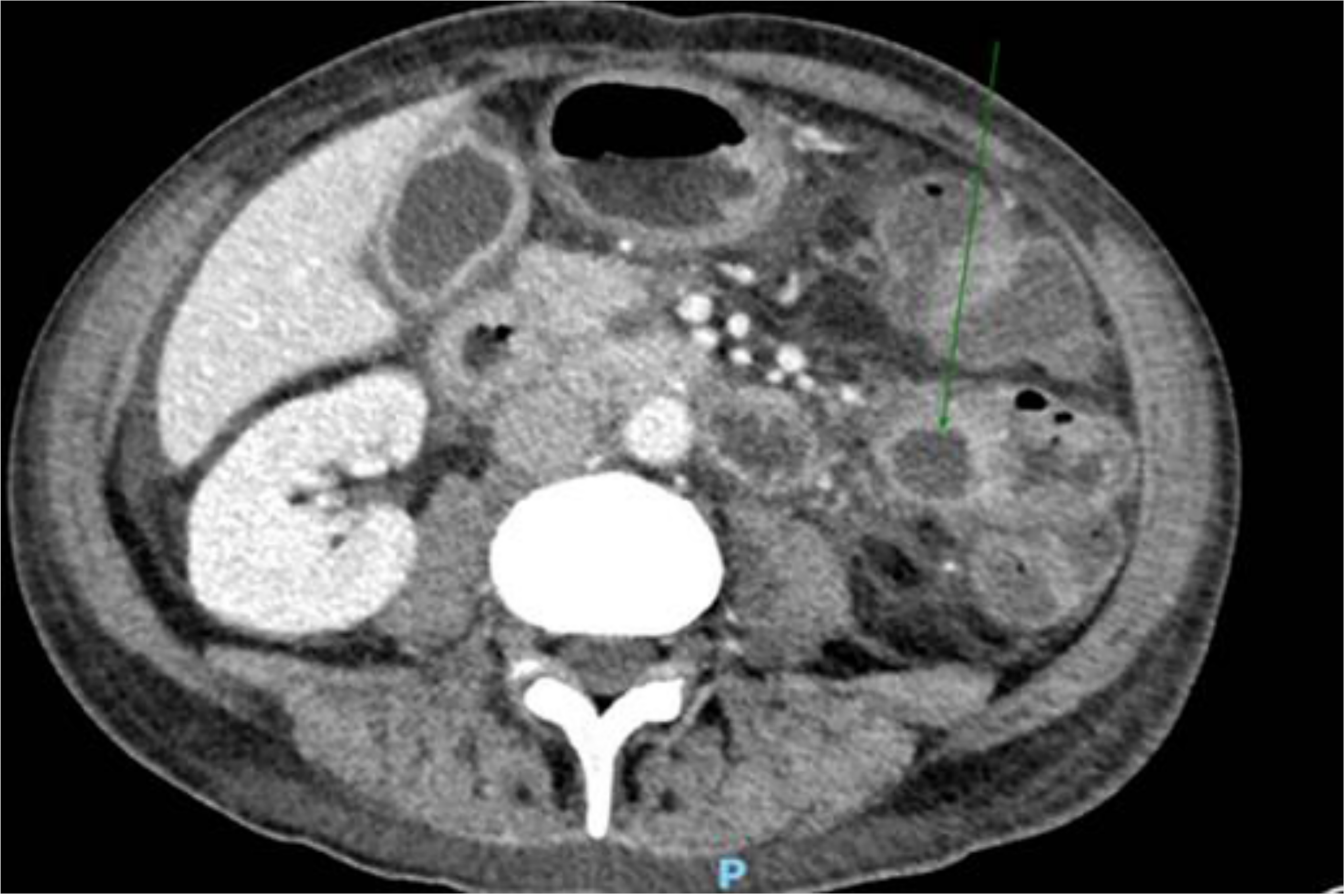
Consistent reduction of the inhomogeneous abdominal masses, as for physiological evolution of pancreatitis in resolution, documented at CT scan.
